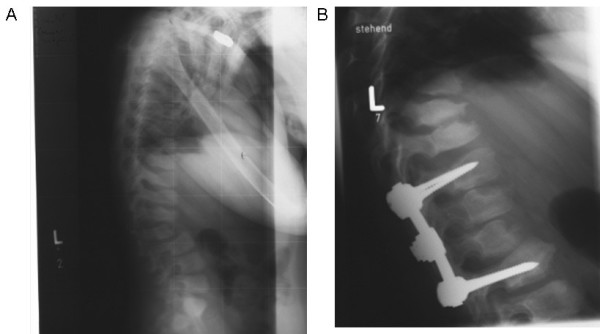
Radiography of kyphoscoliosis in alpha-mannosidosis before and after orthopedic correction. A. Kyphoscoliosis with skeletal abnormalities of all vertebrae. B. Orthopedic correction of kyphosis.
